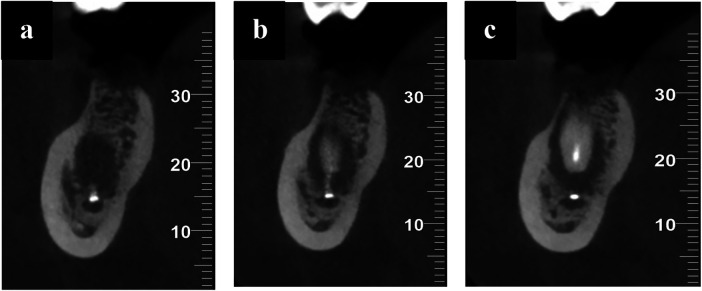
(a–c) sagittal sections from the CBCT scan showing the presence of radiopaque material in the periapical region of tooth 46, extending around and into the right mandibular canal following extrusion of calcium hydroxide.
